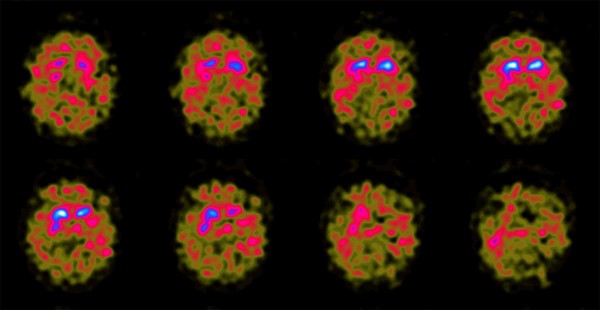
Abnormal DAT-SPECT. [123I]FP-CIT SPECT images showed bilateral and symmetrical reduced tracer uptake in the striatum indicative of nigrostriatal degeneration.
